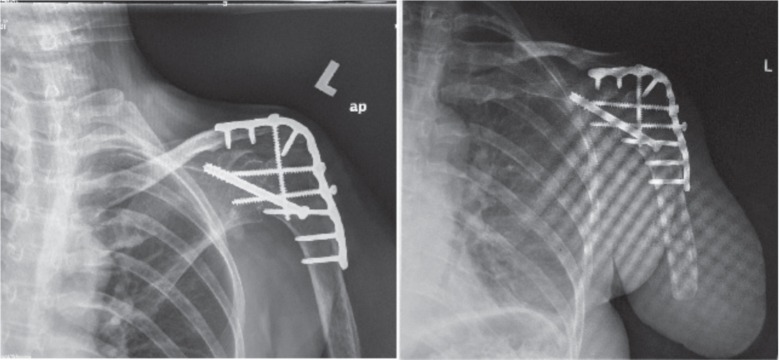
(left) – Plain AP radiographs of left shoulder pre-operatively, showing previous fusion fixation of left shoulder; (right) – Plain AP radiographs of left shoulder post trans-humeral amputation.
